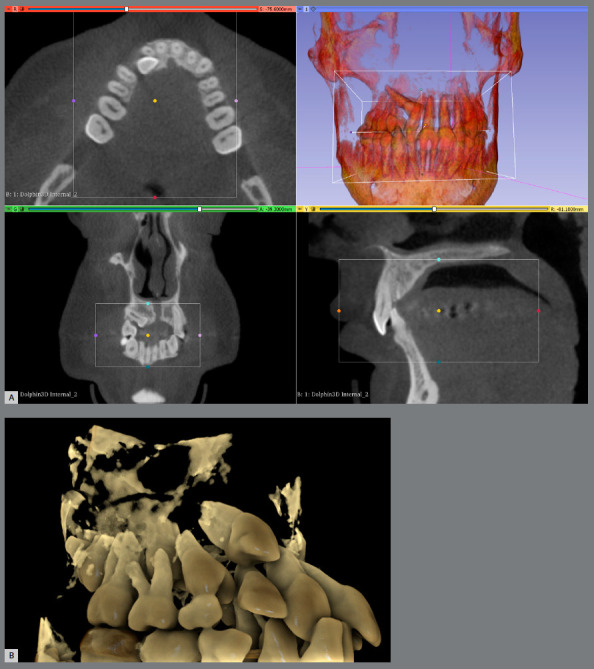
A) CBCT with multiplanar view and 3D rendering. B) Enhanced CBCT image pre-segmentation, using the Drishti software ( The Australian National University, Canberra, Australia ).
